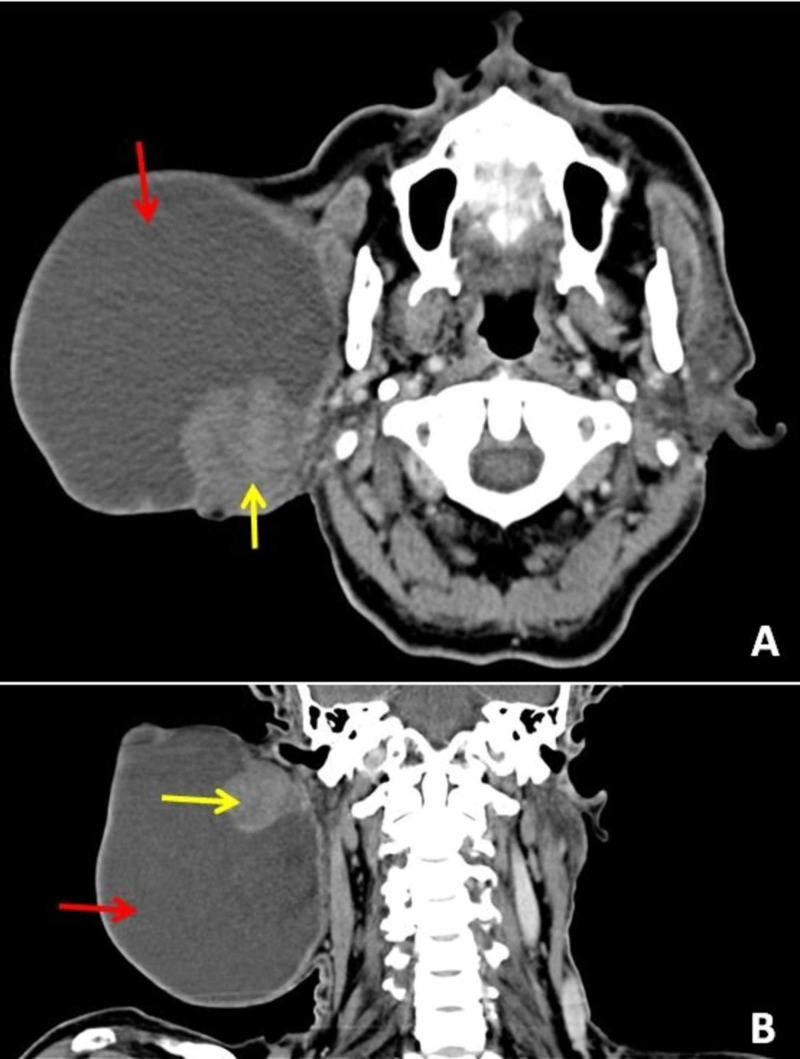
Post-contrast CECT A: Coronal, and B: Axial view showing a well-defined fluid attenuating cystic lesion (red arrow) with an eccentric, enhancing nodular solid component (yellow arrow) in the right parotid region. CECT: contrast-enhanced computed tomography
